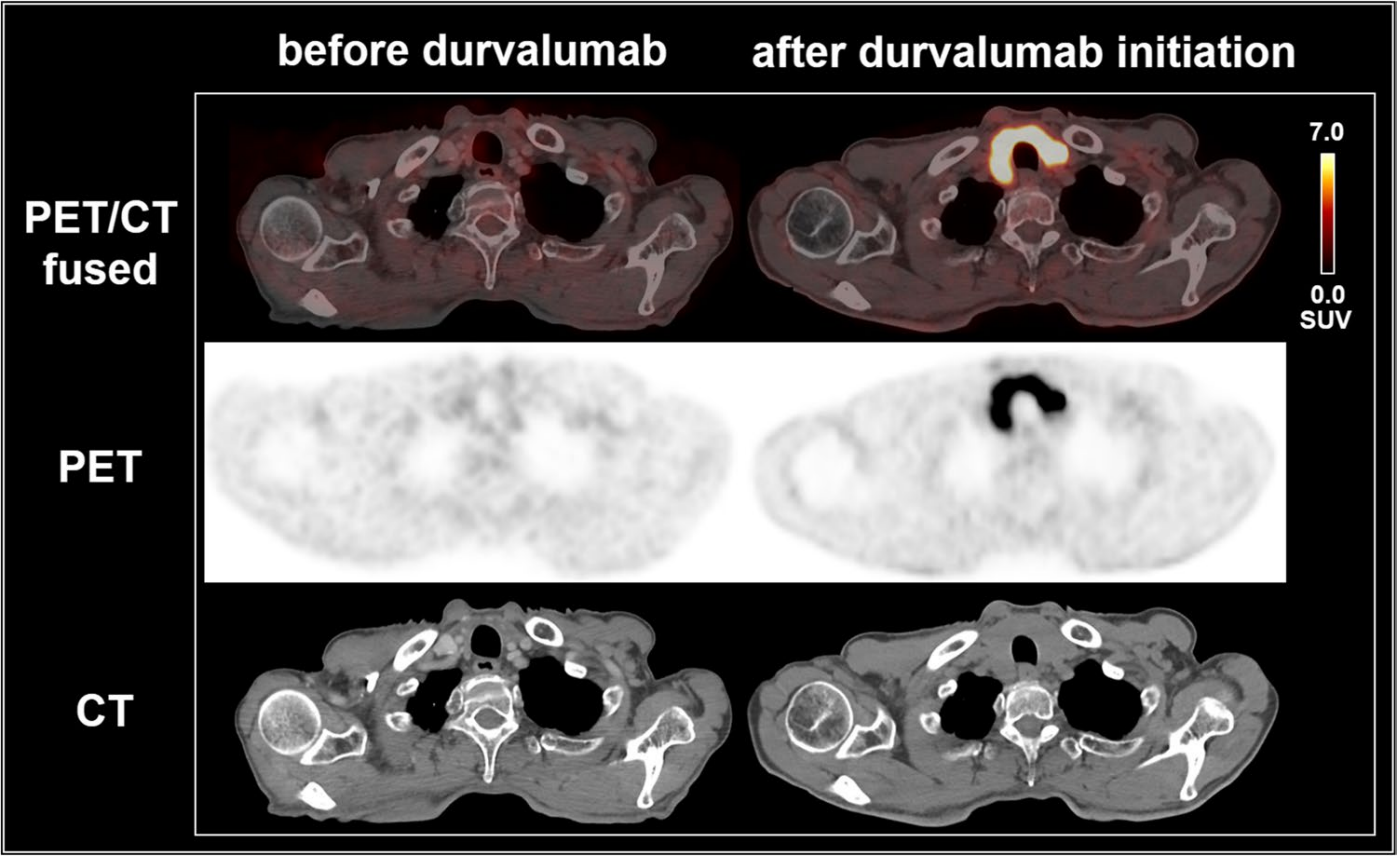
[^18^F]FDG PET/CT scans of a 69-year old man with stage IIIB NSCLC of the right upper lobe, cT3 cN2 cM0, adenocarcinoma, CK7-positive, TTF1-positive, PD-L1: 10%, active smoker (75 py), undergoing CRT with 63.6 Gy, Cisplatin/Vinorelbine, and durvalumab maintenance treatment, who developed an immunotherapy-related thyroiditis. After durvalumab administration, the thyroid gland significantly increased in volume and showed a markedly increased glucose metabolism on [^18^F]FDG PET/CT (SUV_max_ 17.0). The blood tests substantiated the suspicion of an immunotherapy-related thyroiditis with hyperthyroidism (TSH < 0.01 µU/ml) at the day of the PET/CT scan which turned to hypothyroidism 6 weeks later (TSH 56.4 µU/ml, fT4 < 0.3 ng/dl, fT3 < 1.0 pg/ml). During the follow-up time of 24 months the patient did not experience tumor progression
